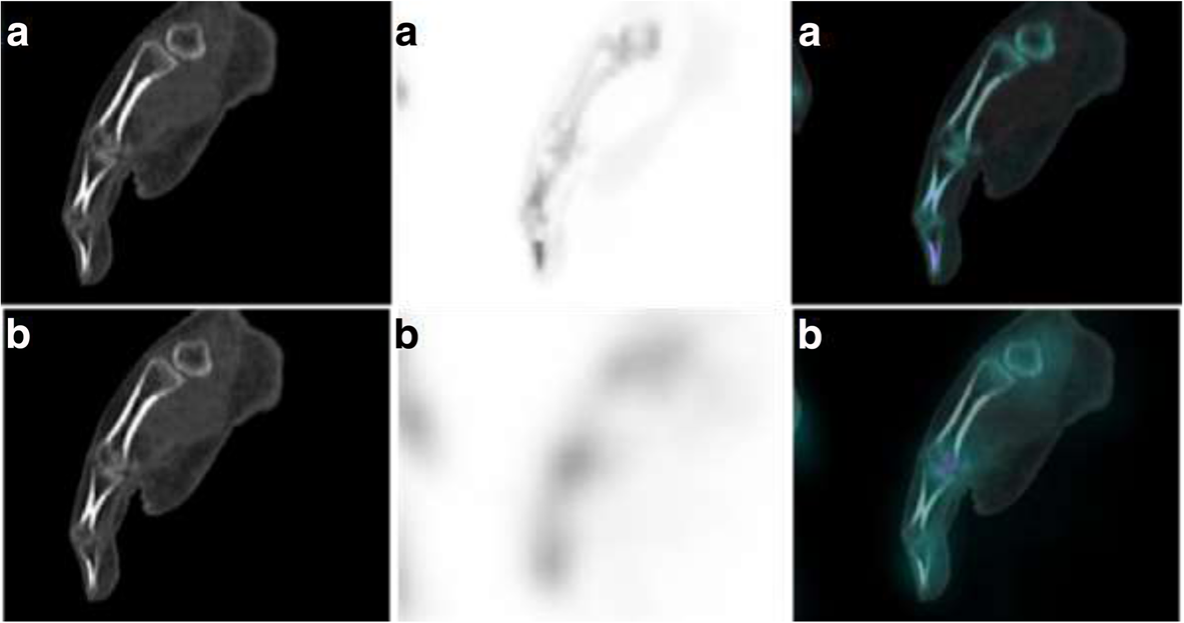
Example of false positive of xSPECT Bone®. This SPECT/CT was performed in order to explore a focal distal thumb pain persisting after a traumatism occurred several months ago. A moderate uptake involving only the last phalange of the thumb, higher than the other phalangeal uptakes, is observed on the xB image (a), matched with the focal pain and suggesting an osteitis. However, there is no pathological uptake on the F3D image (b). The MRI performed after SPECT/CT was normal and ruled out the osteitis. A conversion disorder was diagnosed
